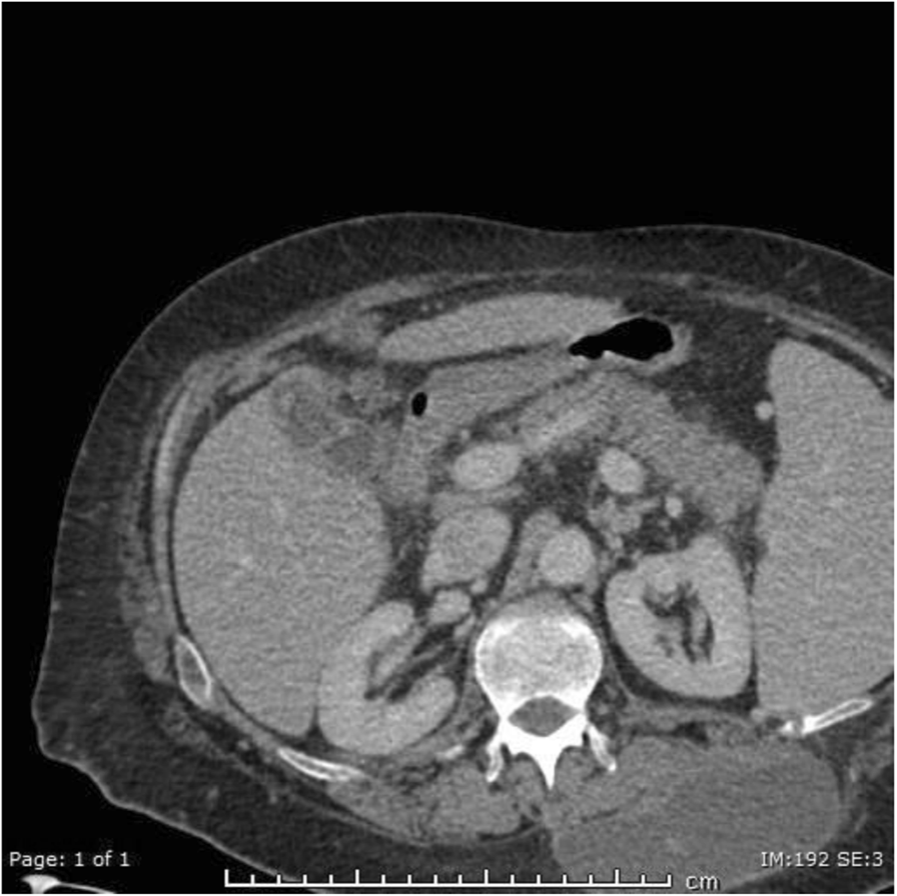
Pre-contrast CT. Gallbladder wall thickening
